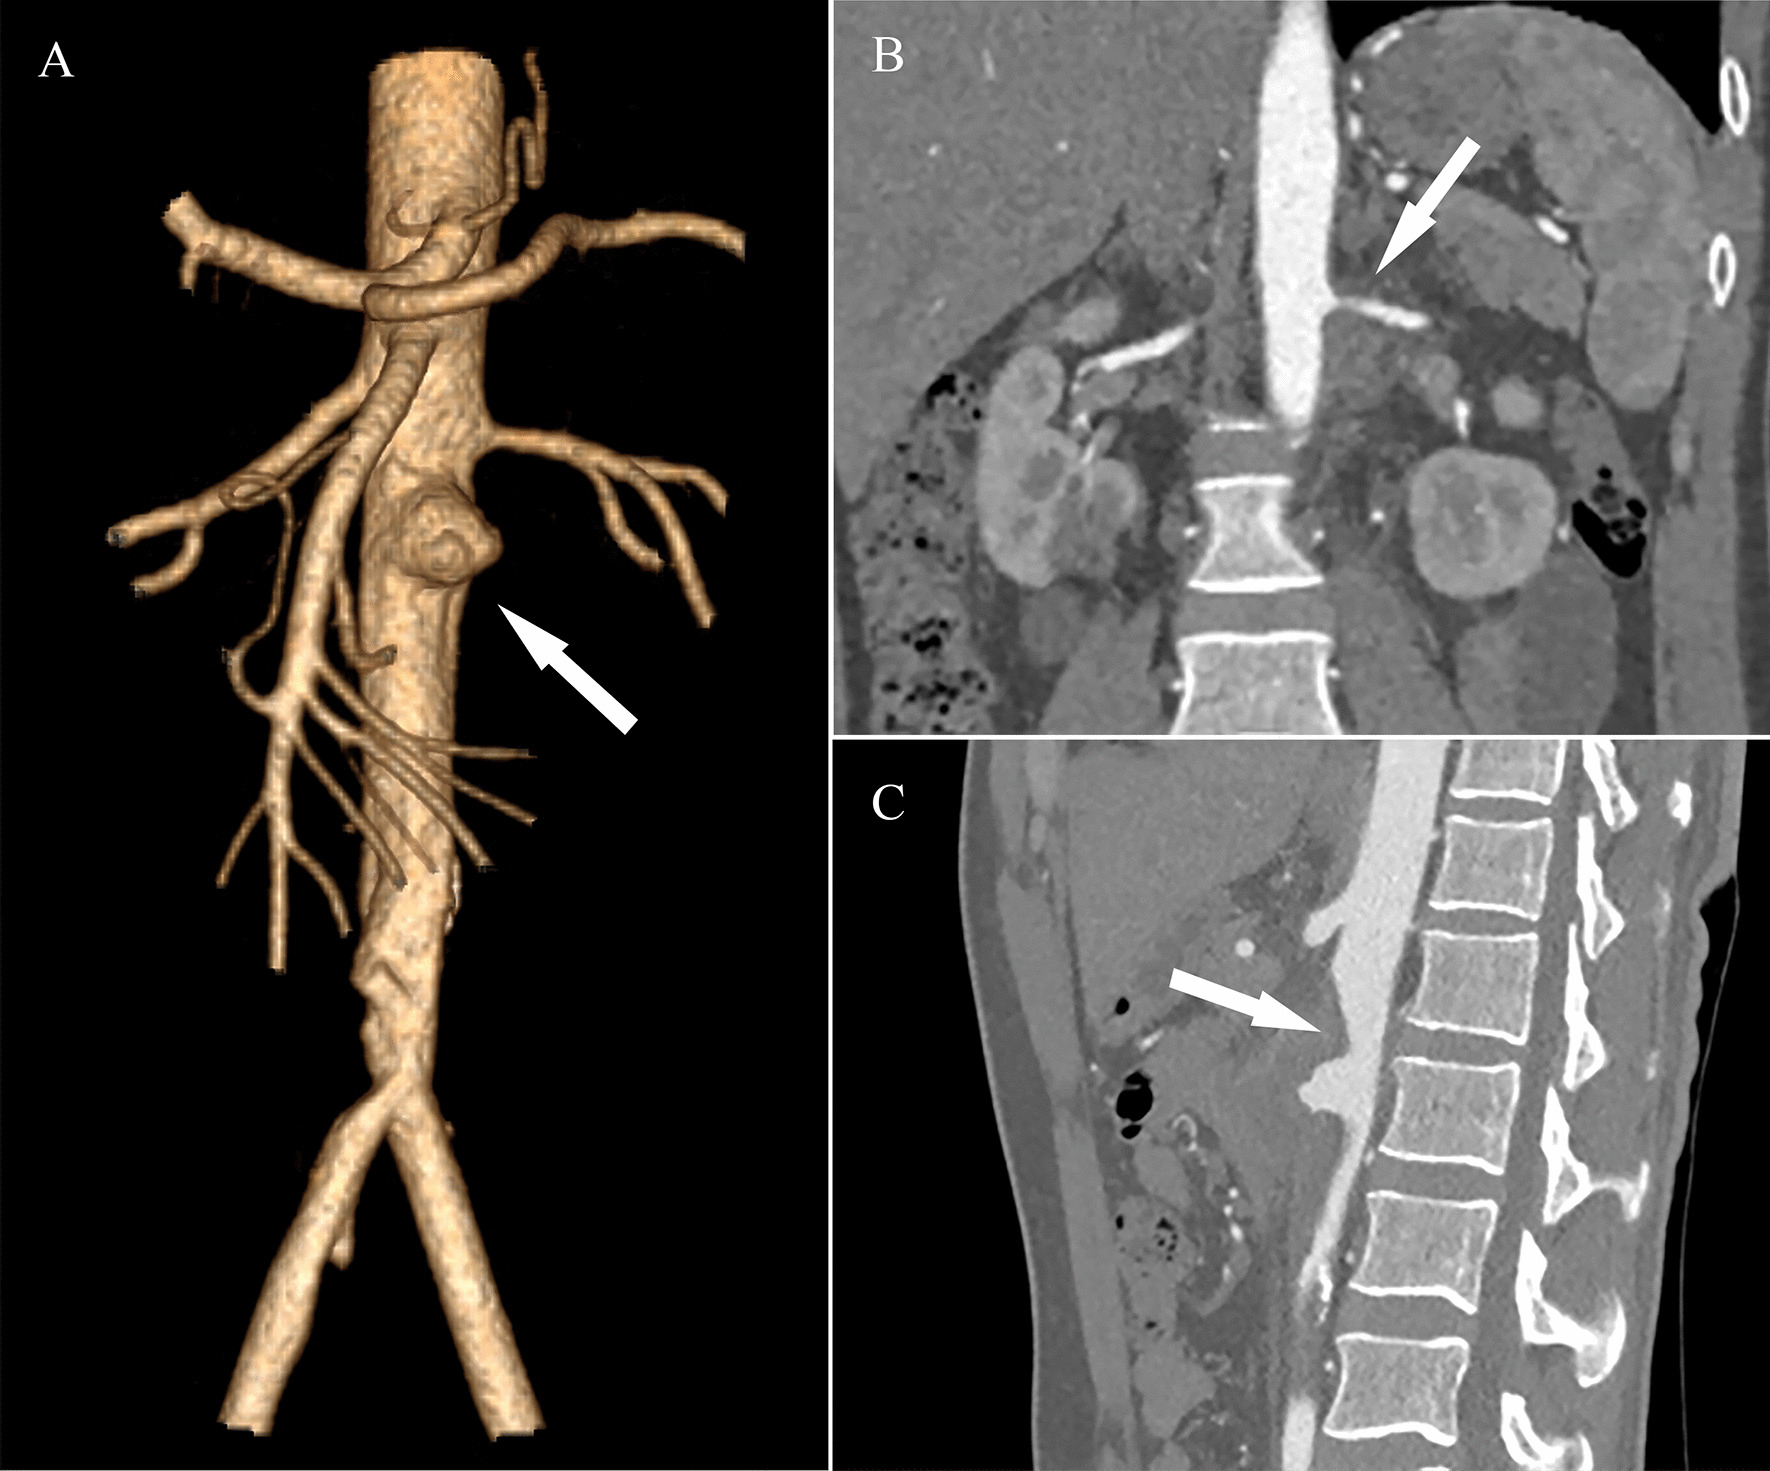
CT scans of the second patient A 3D reconstructions of CT scans shows aneurysm (white arrow); B Compression of the left renal artery and the lumen (white arrow); C Abdominal aorta is compressed and deformed by the aneurysm (white arrow)
